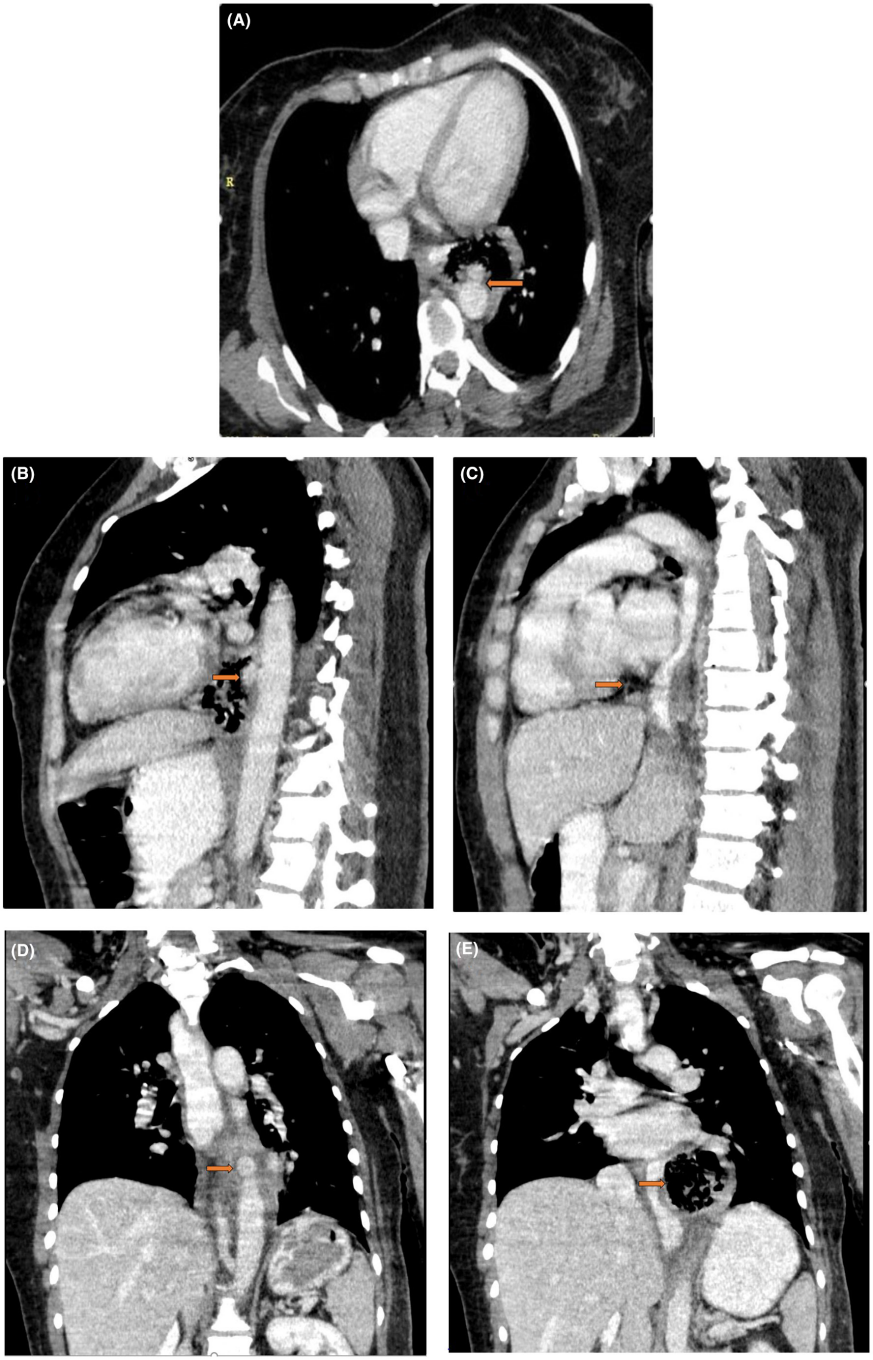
(A, B) Axial and sagittal reconstruction of the CT scan with IV and oral contrast. The images reveal an aortic wall outpouching with gas densities and fat stranding around it (arrow). (C) Sagittal reconstruction of the CT scan, indicating fat stranding and a deviation in the mid‐esophagus (arrow). (D, E) Coronal reconstruction of the CT scan, highlighting an aortic wall outpouching with gas densities and fat stranding around it adjacent to the esophagus (arrow). No obvious contrast extravasation was observed.
